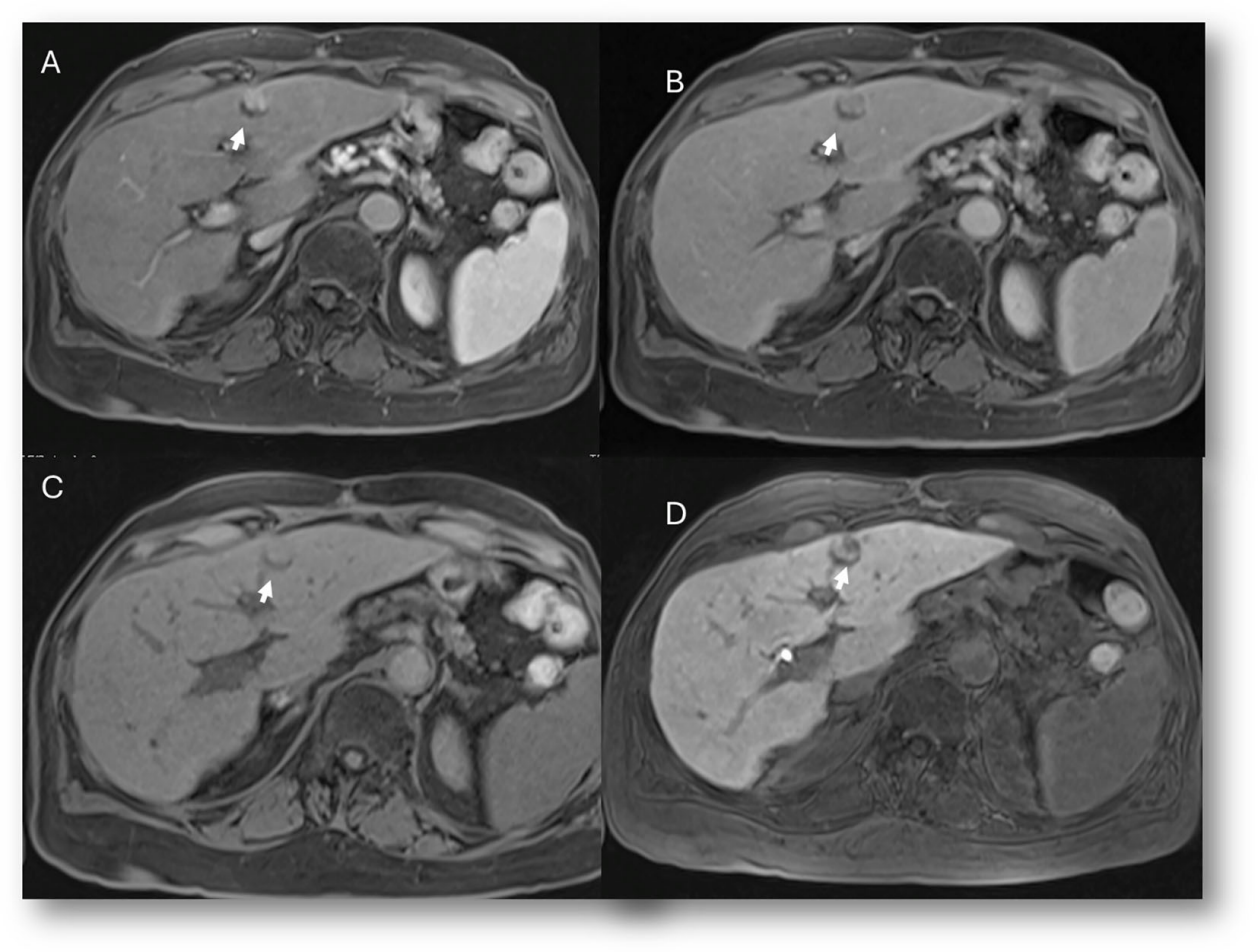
MRI assessment of nodule-in-nodule in early HCC (arrows). Contrast study with specific agent (Gd-EOB-DTPA). In (A) (T1-W FS sequence, axial plane), the lesion shows APHE during arterial phase, while during portal (B) and transitional phases no washout is present. (C) Only the nodule-in-nodule shows hypointense signal in the hepatospecific phase (D).
